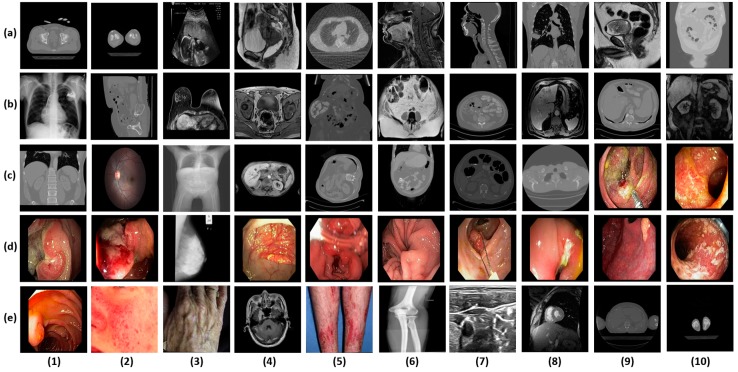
Examples from each class of total 50 classes. (a-1) Hip computed tomography (CT); (a-2) knee CT; (a-3) ultrasound; (a-4) cervix magnetic resonance imaging (MRI); (a-5) 4D lung CT; (a-6) head, neck MRI; (a-7) head, neck CT; (a-8) lung CT; (a-9) bladder MRI; (a-10) bladder CT; (b-1) chest X-Ray; (b-2) urography CT; (b-3) breast MRI; (b-4) prostate MRI; (b-5) uterus CT; (b-6) rectum MRI; (b-7) ovary CT; (b-8) liver MRI; (b-9) liver CT; (b-10) kidney, renal MRI; (c-1) kidney, renal CT; (c-2) retina; (c-3) CT topogram; (c-4) pancreas MRI; (c-5) pancreas CT; (c-6) stomach CT; (c-7) colonography CT; (c-8) esophagus CT; (c-9) malignant tumors; (c-10) sigmoid colon; (d-1) rectum; (d-2) colon; (d-3) breast mammogram; (d-4) caecum; (d-5) duodenal bulb; (d-6) normal esophagus; (d-7) benign tumors; (d-8) Crohn’s disease; (d-9) gastric fundus; (d-10) ulcerative colitis; (e-1) upper endoscopy; (e-2) facial acne; (e-3) hand, foot allergies; (e-4) brain MRI; (e-5) legs, arms allergies; (e-6) bones X-rays; (e-7) neck nerves; (e-8) cardiac MRI; (e-9) shoulder CT; (e-10) ankle CT.
